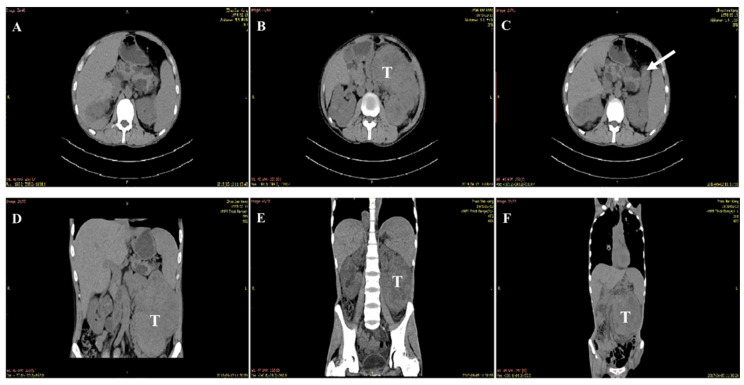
Pathological findings. (A) This image shows a normal transverse CT scan. (B) This transverse CT scan shows a large mass lesion with low-density cystic lesions (T), arising from the left kidney. (C) This transverse CT scan shows an aberrant extension of the left kidney (white arrow). (D–F) Coronal CT scans show that the RCC originated from the left kidney and they also provide information regarding tumor size.
